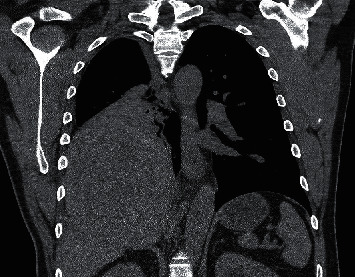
CT scan of the chest on presentation to hospital revealing very large (14 × 14 × 12 cm) lung mass.
